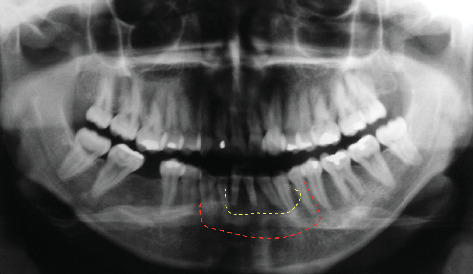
Preoperative panoramic radiograph showing the lesion (yellow dashed line) and planned surgical approach (red dashed line).
